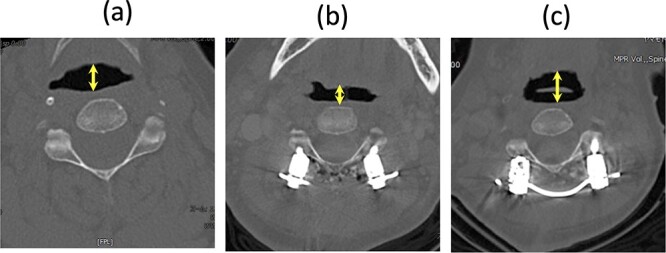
CT axial image at the C2 level in a supine position; (a) before the second surgery, (b) after the second surgery, and (c) after the third surgery; the arrow shows the pharyngeal diameter.
